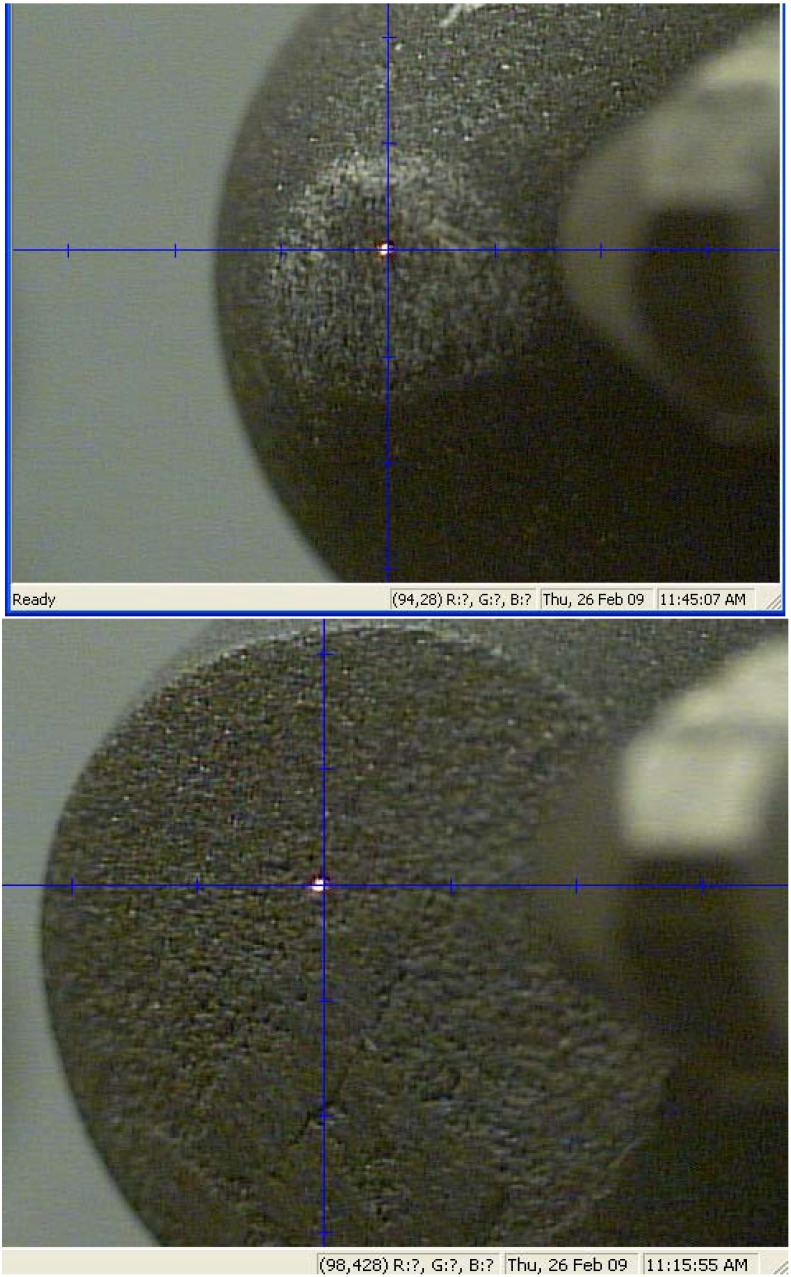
Optical images of both samples as mounted in the sample holder of GADDS^TM^. Upper image is of the high quality TSDC (*i.e.,* high wear resistance, sample 1) with a small wear flat (upper) and the lower image of poor quality TSDC (*i.e.,* low wear resistance, sample 5) showing the large wear flat. The bright laser spot on each of the wear surfaces indicates the location of the x-ray beam used in the analyses (Magnifications: upper image ×40, lower image ×30).
